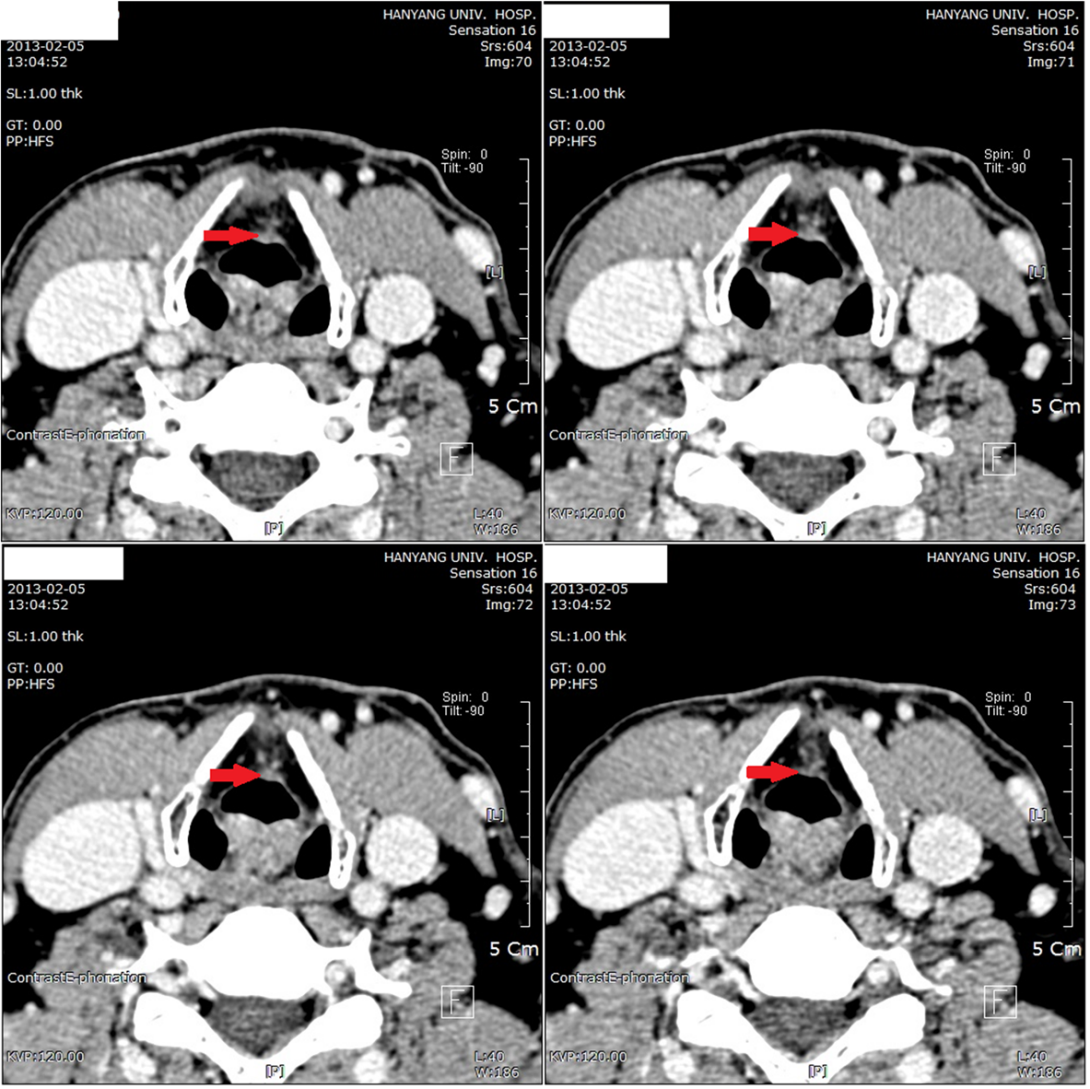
Neck 3-D CT/c CE (02/05/2013). It showed that the lesion had invaded the anterior commissure of the larynx but was limited to the right vocal cord with no invasion around the glottis
